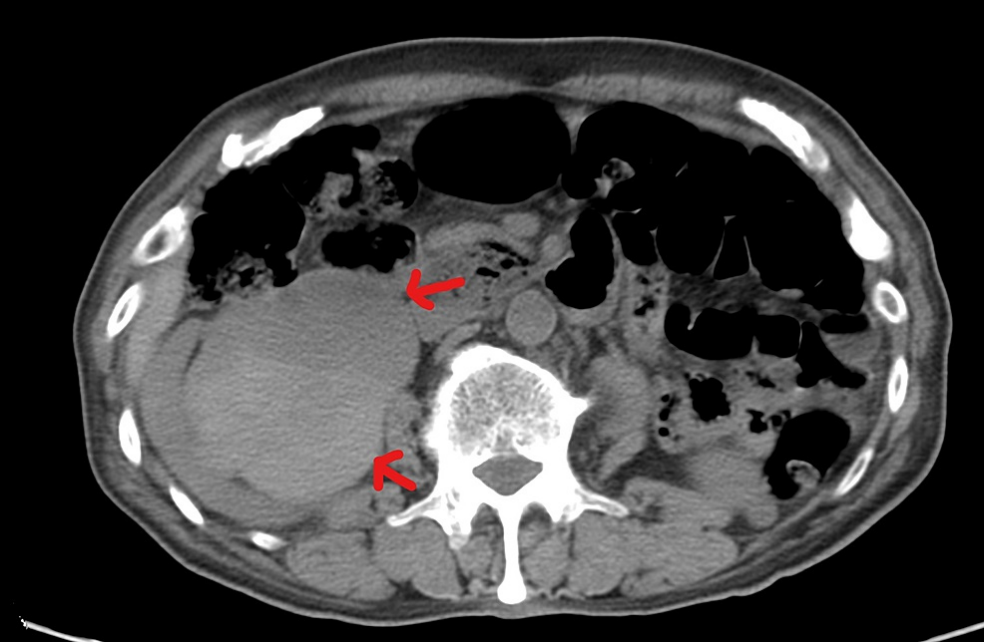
Axial CT scan through the level of the right kidney demonstrating a massively dilated right renal pelvis with large contained hematoma (red arrows).
